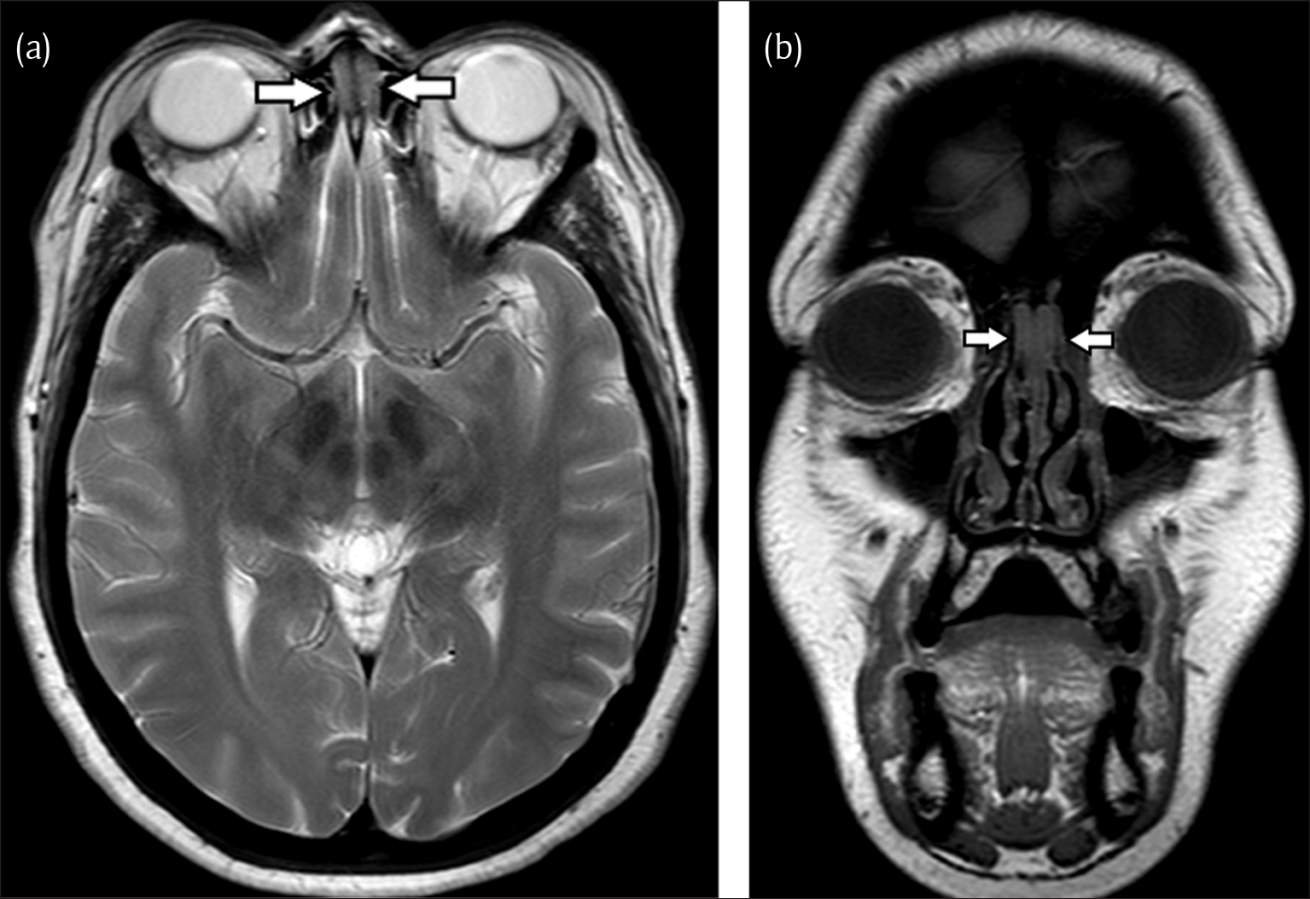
(a) On axial T2-weighted images and coronal T1-weighted (b) MR images the bilateral mass (arrows) appears iso-intense to white matter. The lesions are sharply delineated and do not involve or cross the cribriform plate.
